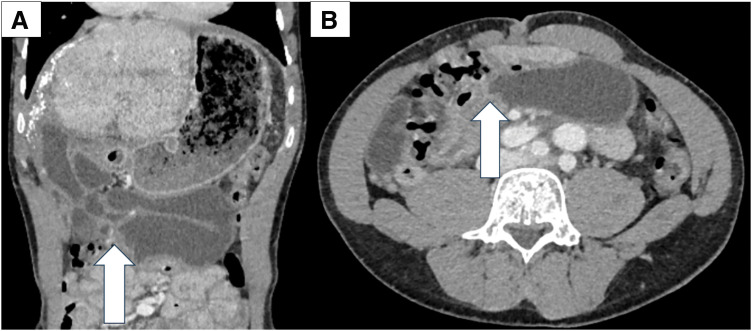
Preoperative CT image. (A) Dilatation of the afferent limb with beak sign was observed in coronal view. (B) Axial view. White arrow: Beak sign.
